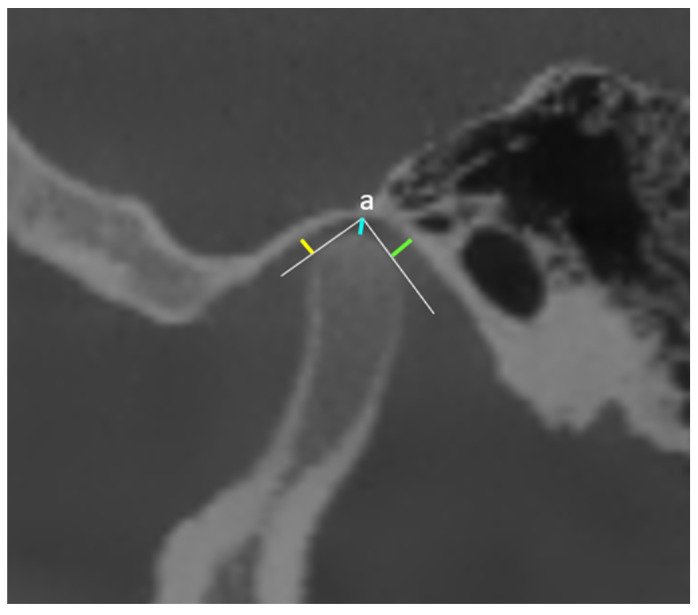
Sagittal CBCT image demonstrating the measurements of AJS (yellow line), SJS (blue line), and PJS (green line).
